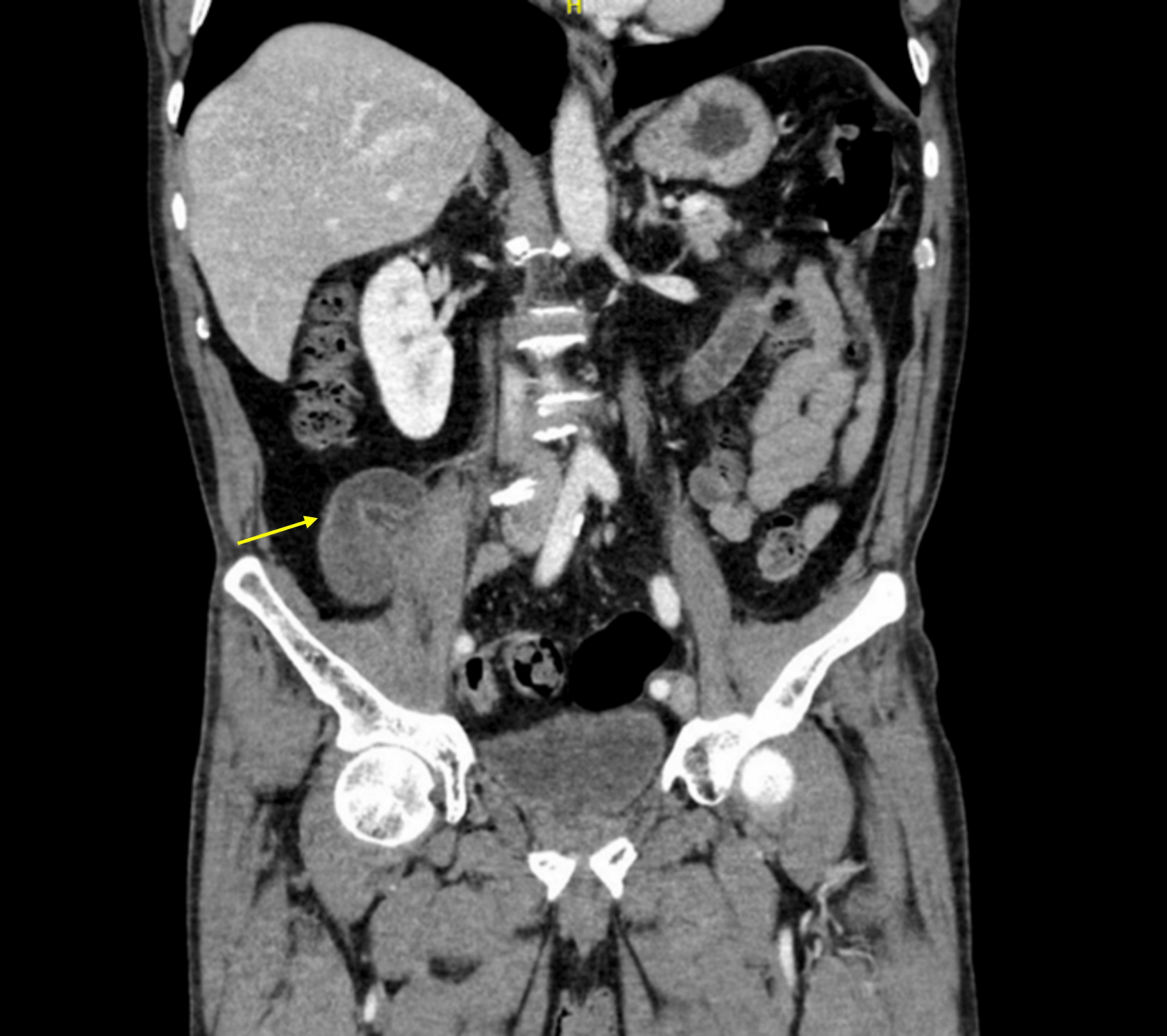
Coronal section of computed tomography imaging showing distended appendix (arrow).
